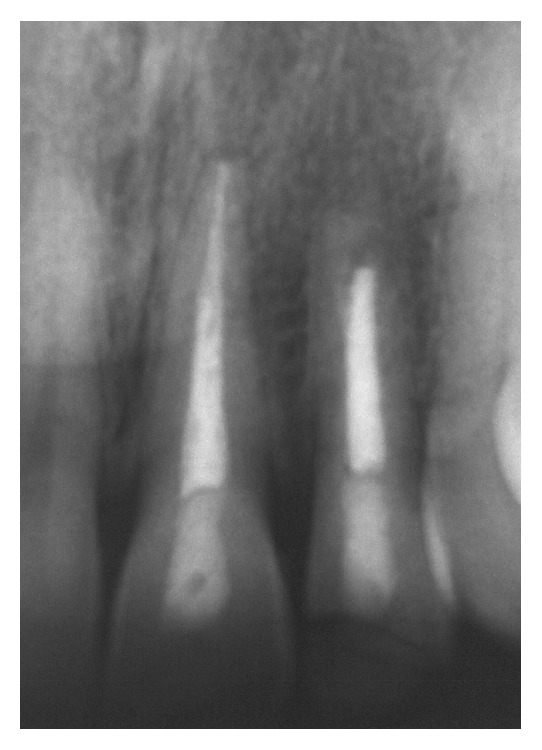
Radiograph of thirteen-year follow-up (March 2012).
